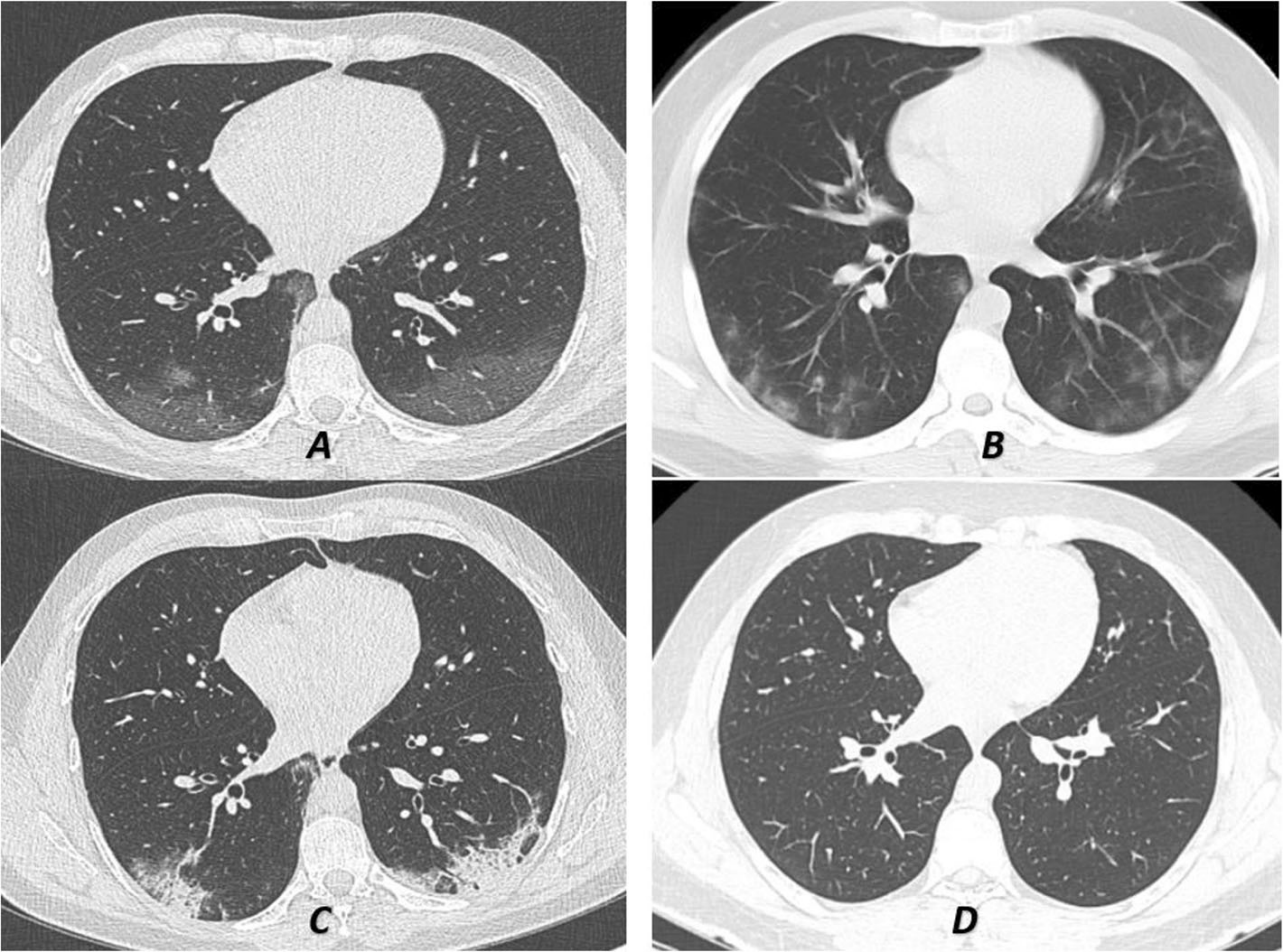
A 27-year-old medical staff who contacted a COVID 19 positive patient and complained of dyspnea and fever. He was diagnosed to have COVID-19 confirmed by a positive RT-PCR test. The 1st CT (lung window) was done 2 days after the onset of the symptoms (a) revealed the presence of a bilateral lower lobar posterior and peripheral ill-defined veiling of ground-glass opacity. Follow-up CT (b) after 7 days from the onset of symptoms showed multi-lobar subpleural more defined multiple ground-glass patches which denoted disease progression. Peripheral subsegmental consolidative patches with fewer areas of ground-glass opacity were noted in (c) which was done after 12 days from the onset of symptoms (stage 3). (d) represented CT at the 4th stage after 18 days from the onset of symptoms revealed complete resolution of the previously noted features (negative CT)
